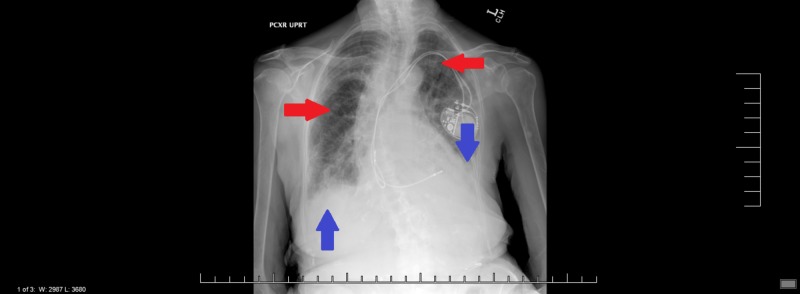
Chest X-ray Diffuse bilateral coarse patchy interstitial infiltrates and pleural effusions Red arrows - interstitial infiltrate, blue arrows - pleural effusions
